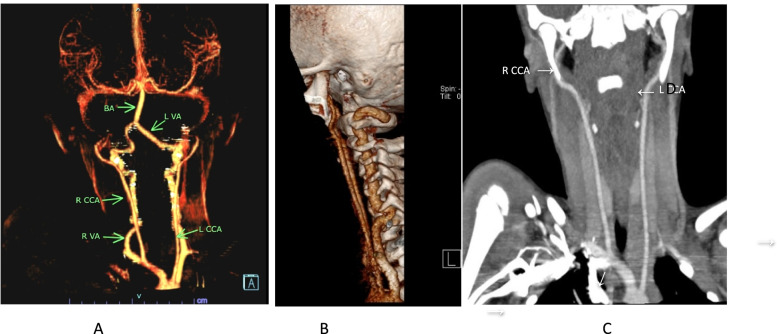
The complete absence of all segments of the internal carotid arteries on either side has shown in VR (A) and dominant vertebral arteries (B) while slim gracile common carotid arteries presented on coronal MIP CTA image (C)
